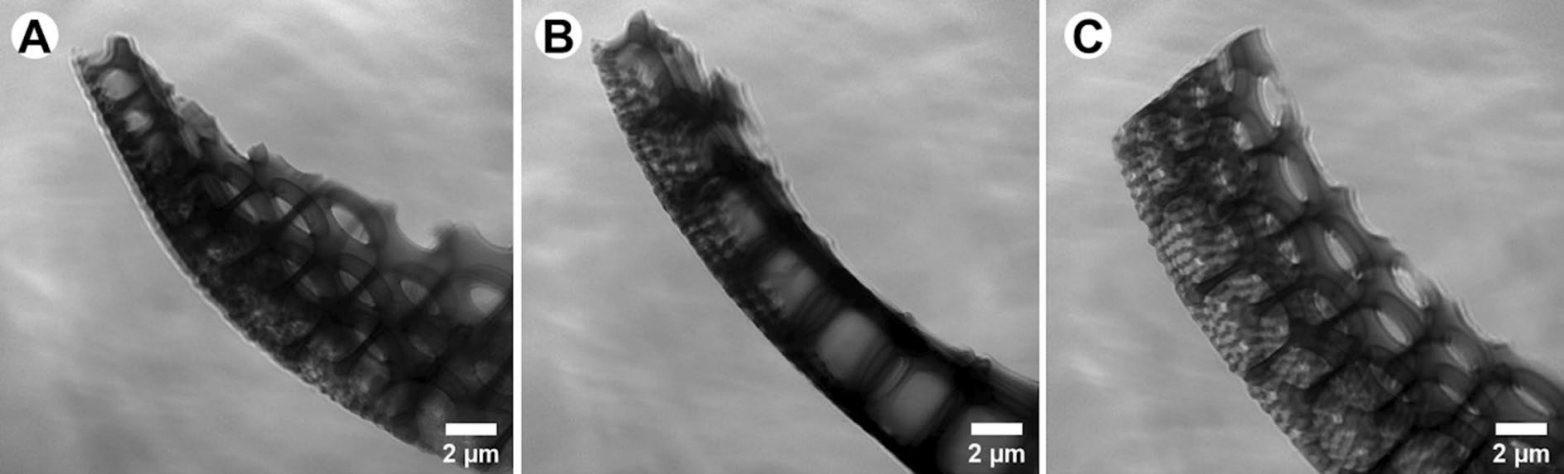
Images of diatoms frustule *Coscinodicus* sp. obtained by soft X-ray nano tomography investigations.
